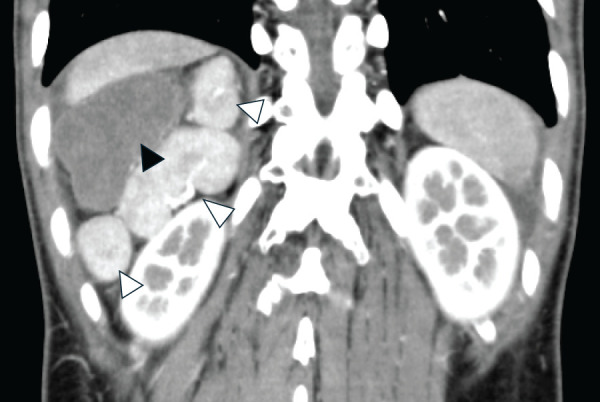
Multiple spleens on CT. In the right upper quadrant of the abdomen, 3 enhanced small spleens are visible (white arrowheads), along with 1 non-enhanced spleen (black arrowhead).
